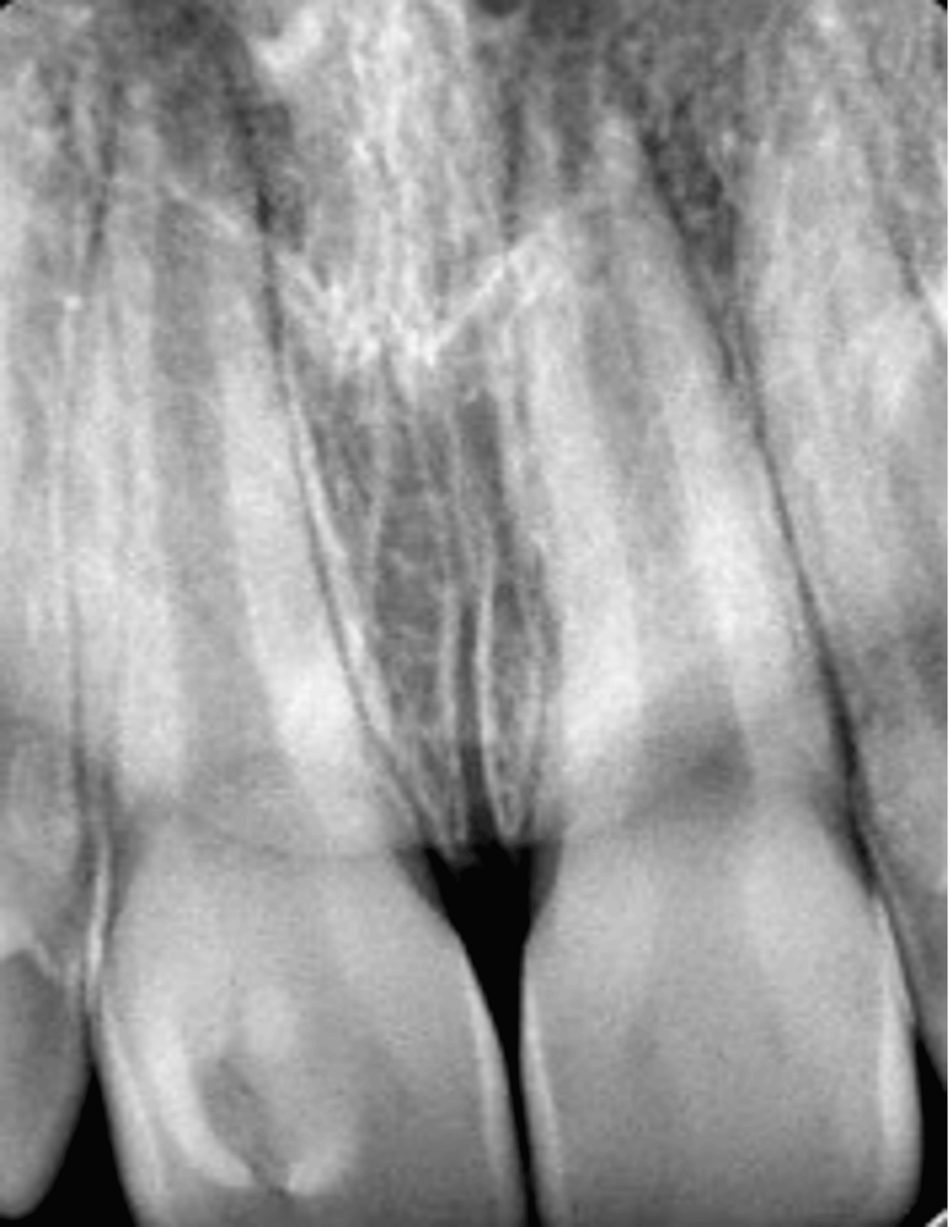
Periapical radiograph showing talon cusp on tooth #11
